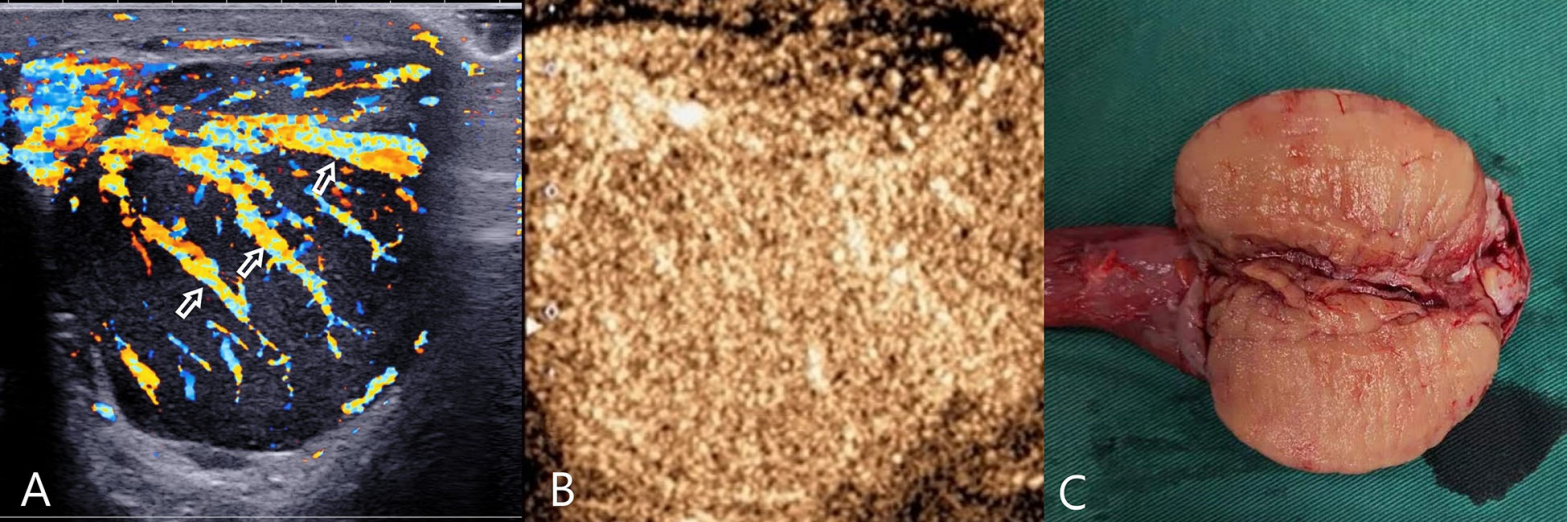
Testicular lymphoma. (A) CDFI: hypoechoic mass in the left testis, abundant blood flow, with multiple perforating vessels(arrow); (B) CEUS, CEUS showed uniform and high enhancement, and no obvious necrotic area was found. (C) Macro-section, Mass occupying the entire testicle; CDFI, Color Doppler Flow Imaging; CEUS, contrast-enhanced ultrasonography.
